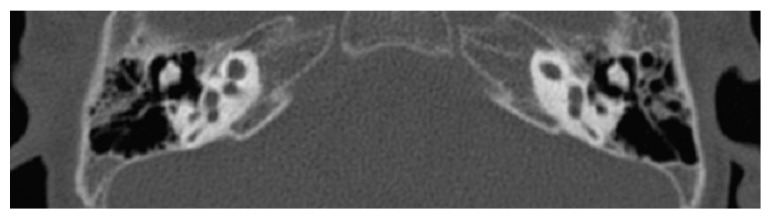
CT imaging of a bilateral incomplete partition type 1. The anterior (cochlear) and posterior (vestibular) parts of the inner ear are clearly distinguishable, with the cochlear portion being cystic and almost normal in terms of size.
